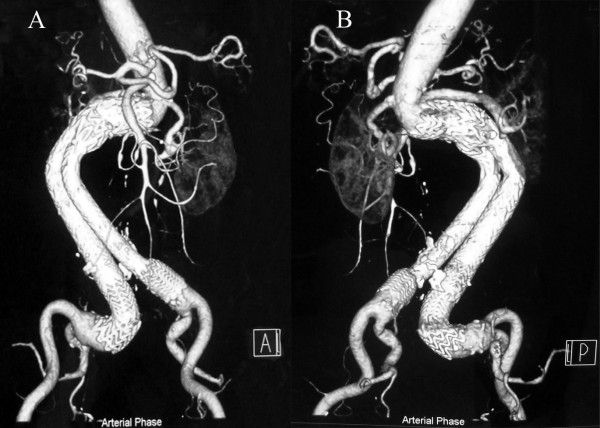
Anterior (A) and posterior (B) view of CT reconstruction at 6-month follow-up shows fluent celiac and bilateral renal arteries.
